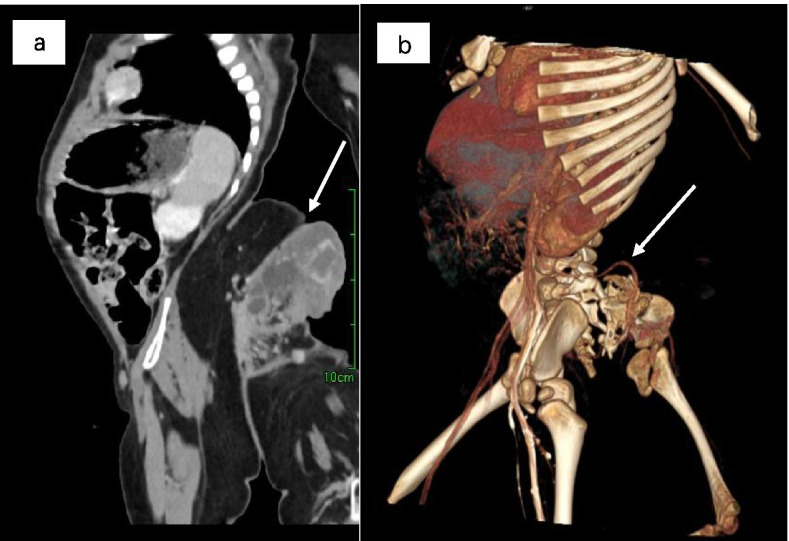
a and b sagittal and 3D CT images showed a large, complex mass with bony structure and blood supply from the aorta of the boy (arrow)
